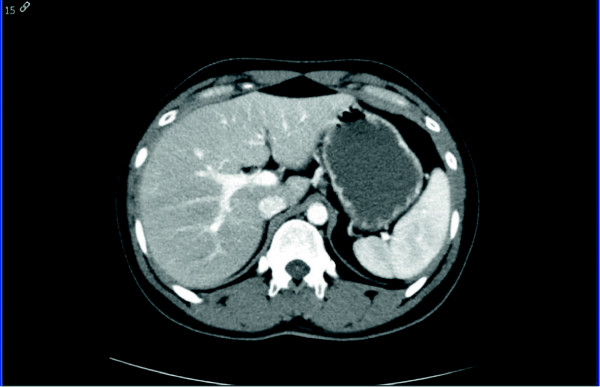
Post-treatment follow-up computed tomography. Scan shows complete resolution of the patient’s peri-portal lymphedema (lower panel).
